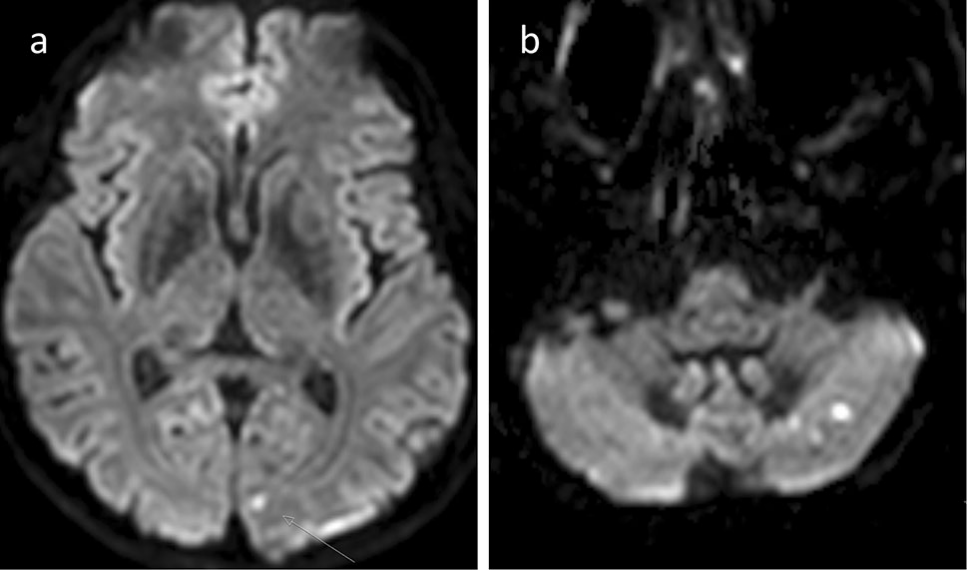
Typical appearance of microembolic brain lesions. a Diffusion-weighted imaging (DWI) of subject No. 2. A typical bright round-shaped diffusion-restricted lesion in the left cuneus. b DWI of subject No. 77. Three lesions of typical round-shape and different sizes in close proximity in the left cerebellar lobe
